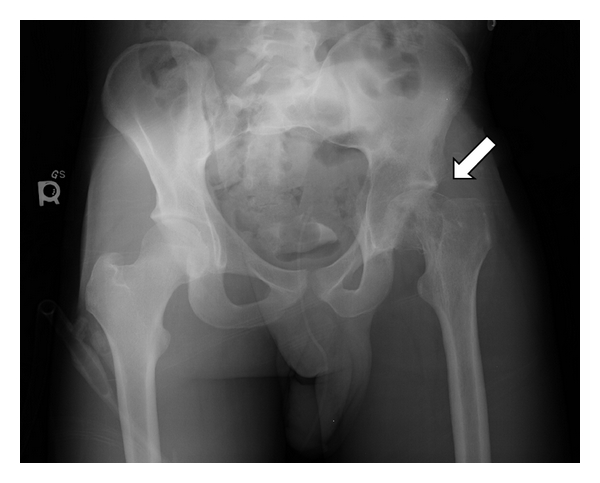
X-ray of the pelvis (anterior-posterior) showing pathological fracture of the left femur neck (arrow).
